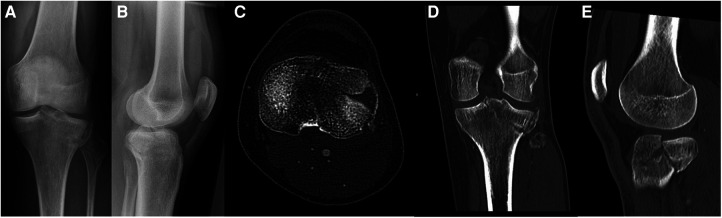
Case 4, 30 year male patient, syncopal fall. Posterolateral split impression fracture, AP (A) and lateral (B) view, axial CT cut (C), coronal (D) and sagittal (E) reconstruction (Schatzker IV, AO 41-B3, Moore Type II lateral, posterolateral 2-column fracture, segments involved: ALL, PLL, PLC, AC, PC).
